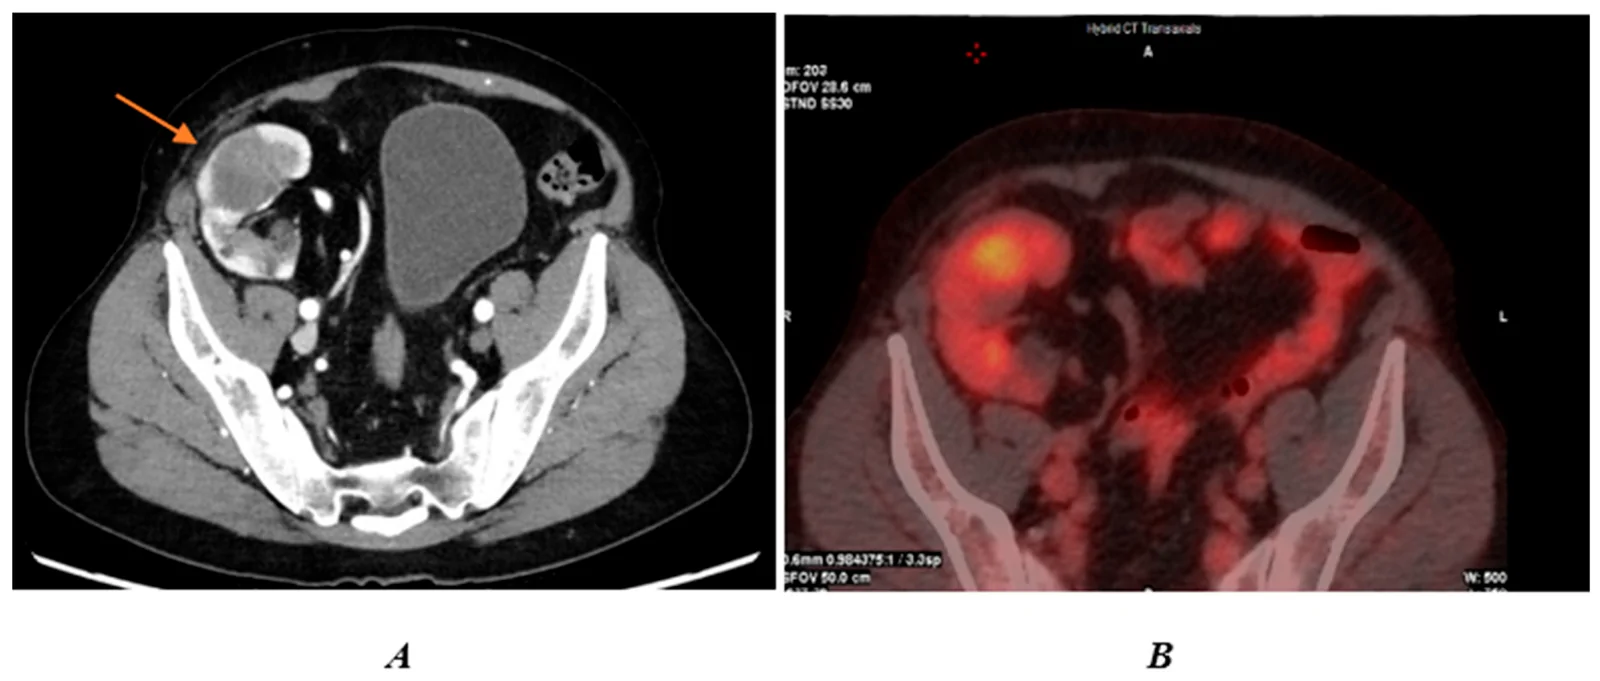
Multimodality imaging of papillary renal cell carcinoma in the kidney allograft prior to treatment. (A) Contrast-enhanced CT depicting the allograft lesion before CT-guided cryoablation. (B) ^18F-FDG PET/CT showing focal increased metabolic activity within the same lesion.
